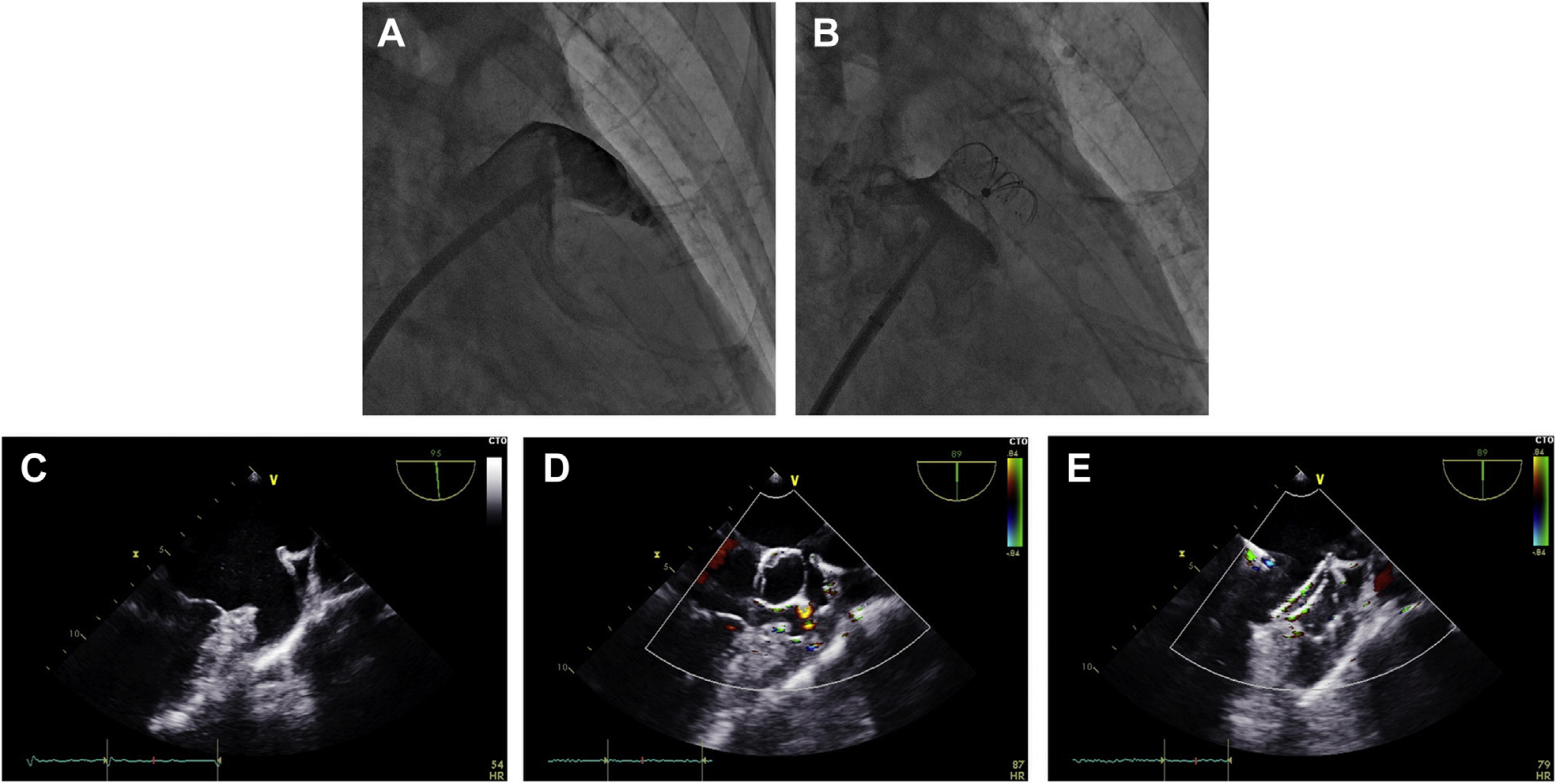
Well-Positioned Device in the LAA (A) LAA angiography before closure. (B) LAA angiography after closure. (C) Transesophageal echocardiography (TEE) examination before LAA closure. (D) A gentle tug test was performed to insure device stability. (E) TEE examination after LAA closure. Angiographic and TEE examinations suggested that the device was stable and completely occluded the LAA. Abbreviation as in Figure 1.
